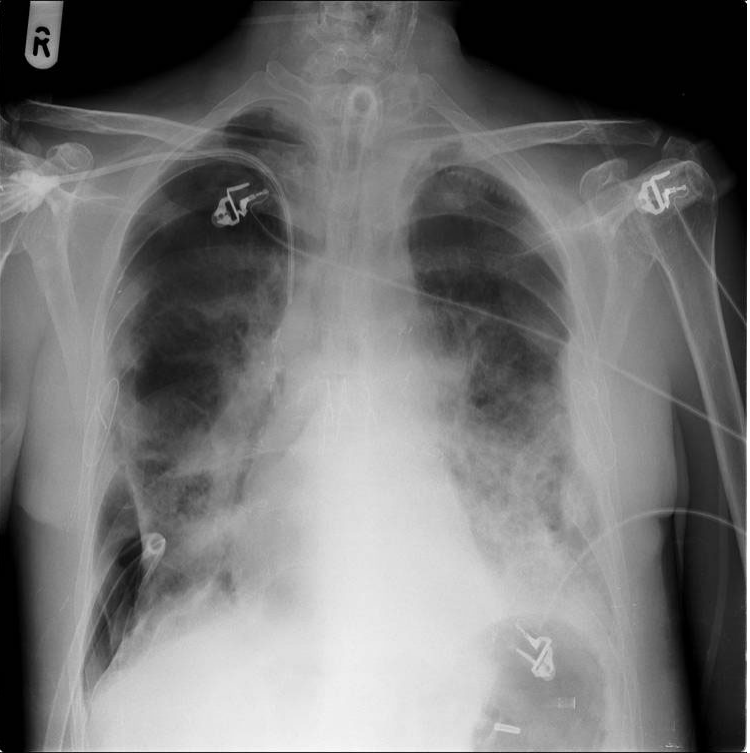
Chest X-ray showing right pneumothorax with an intercostal drain in situ.
